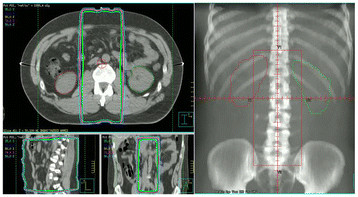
Isodose distributions and antro-posterior digitally reconstructed radiograph of a typical PA field.
